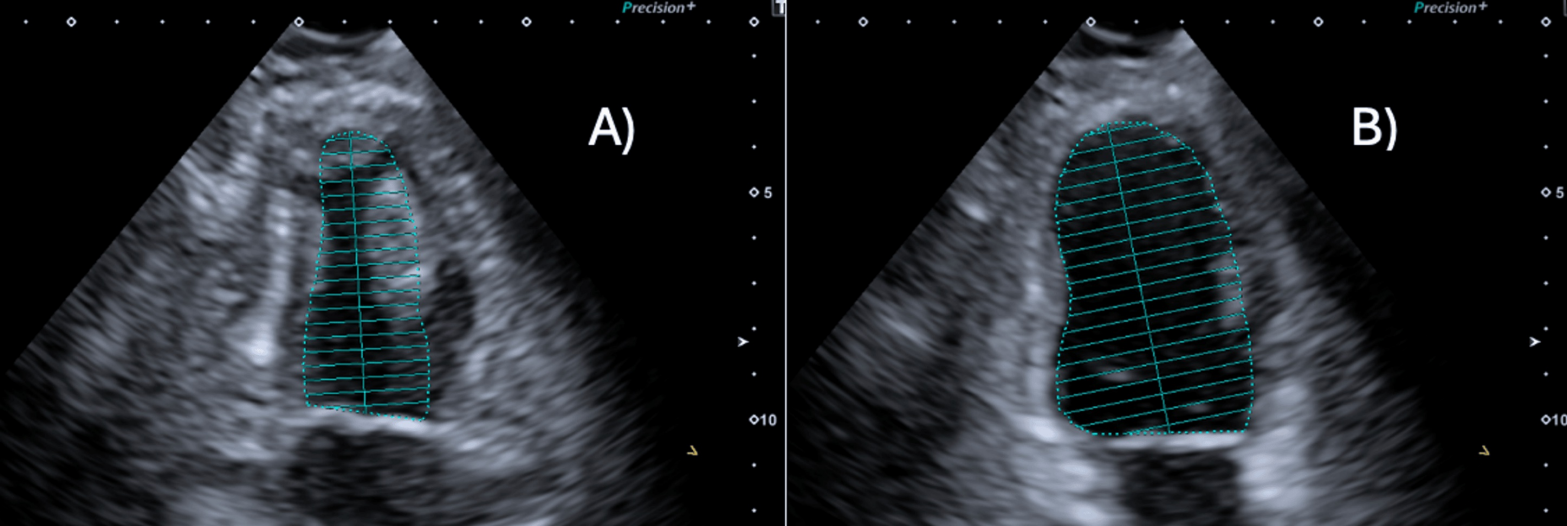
Images of the echocardiography done prior to the procedure showing an overall computed ejection fraction of 65%. A) Image of the left ventricle in full systole. B) Image of the left ventricle in full diastole.
